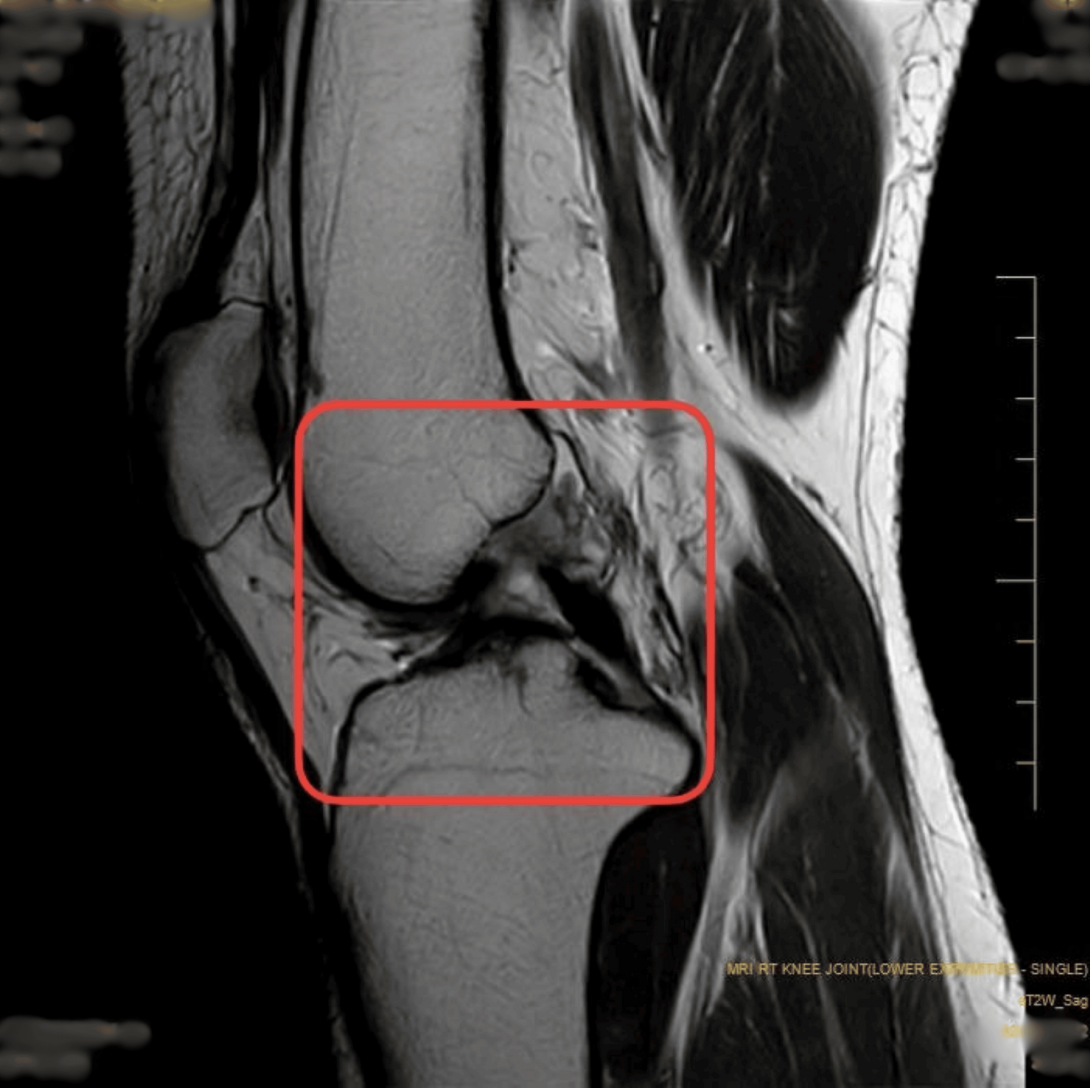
MRI of the right knee suggests a partial tear of the anterior cruciate ligament (ACL) with associated ligamentous strain.
